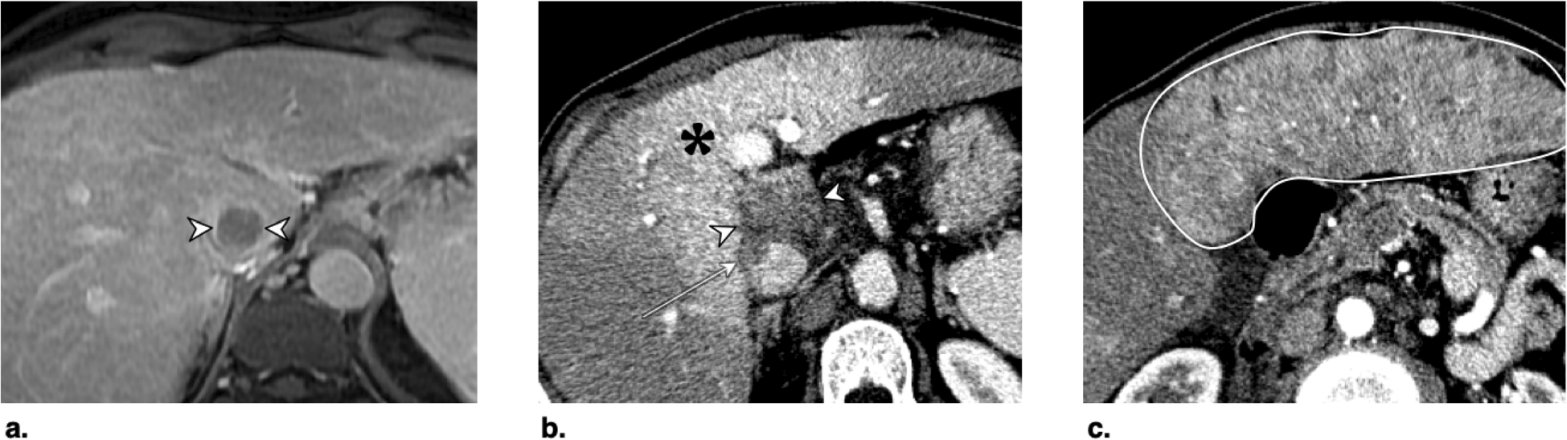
a Axial T1-weighted MR in portal venous phase obtained pretreatment: image shows washout of a 2.5 cm LR-5 observation in caudate lobe (arrow). b Axial CT in late arterial phase (AP) obtained 3 months post stereotactic body radiotherapy (SBRT): image shows tumor progression (arrowheads) and perfusional anomalies related to edema (asterisk). Mural thrombus in inferior vena cava is noted (arrow). The treated observation is categorized LR-TR viable. c Axial CT in late AP obtained 6 months posttreatment: image shows diffuse tumoral infiltration of left lobe (arrow)
